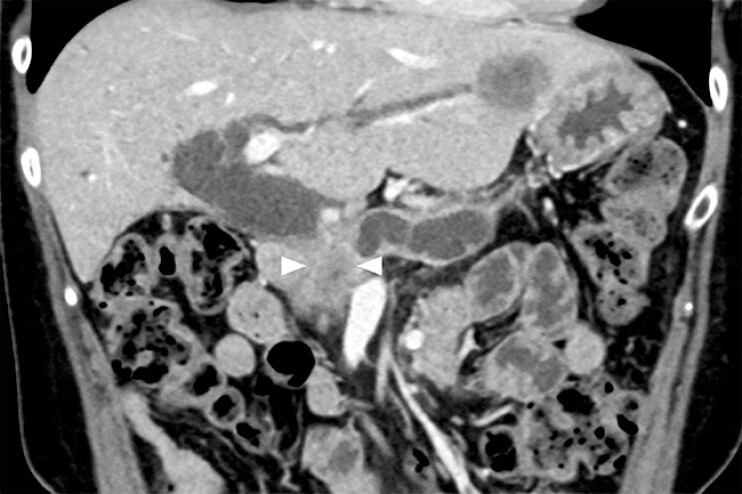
Computed tomography of a 64-year-old woman with unresectable pancreatic cancer and biliary obstruction (arrowheads) revealed liver metastasis in the left lobe.
